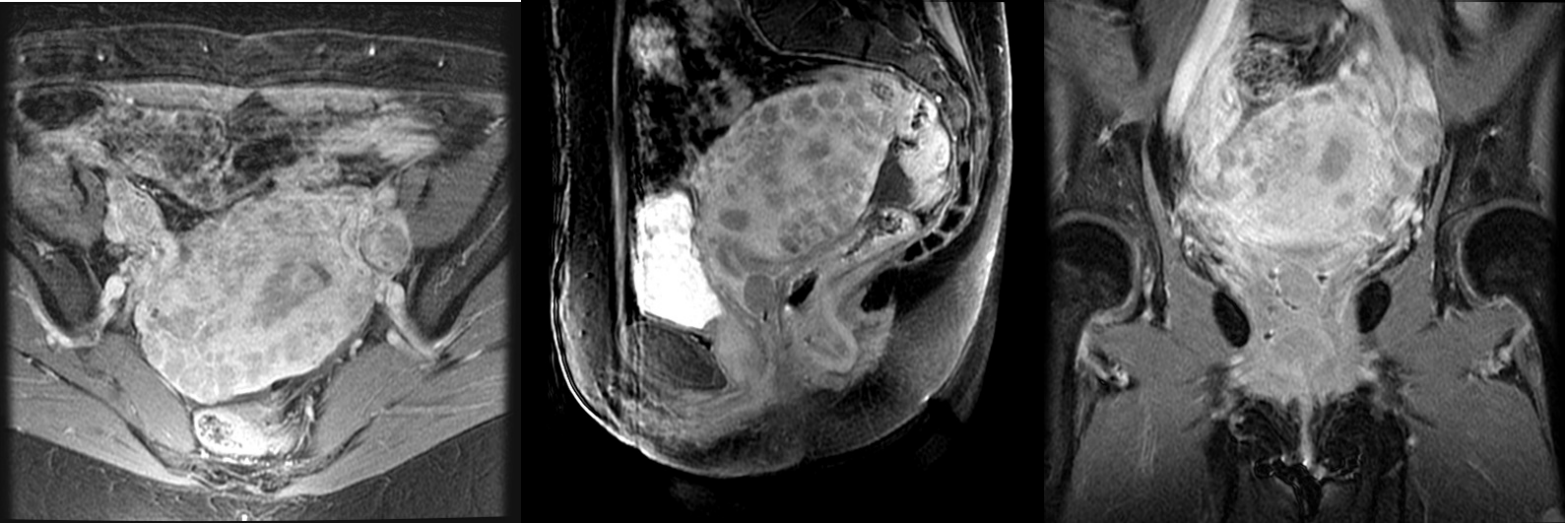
MR imaging at the time of presentation showing bulky, locally advanced disase. Axial, sagittal, and coronal images at the time of diagnosis showing an enlarged uterus measuring 9.6 x 8.6 x 7.7 cm with a polypoid endometrial mass measuring 2.0 x 1.6 cm and an endometrial stripe up to 1.8 cm. Extension to the lateral sidewall was noted bilaterally as well as extension to the lower uterine segment and upper cervix.
